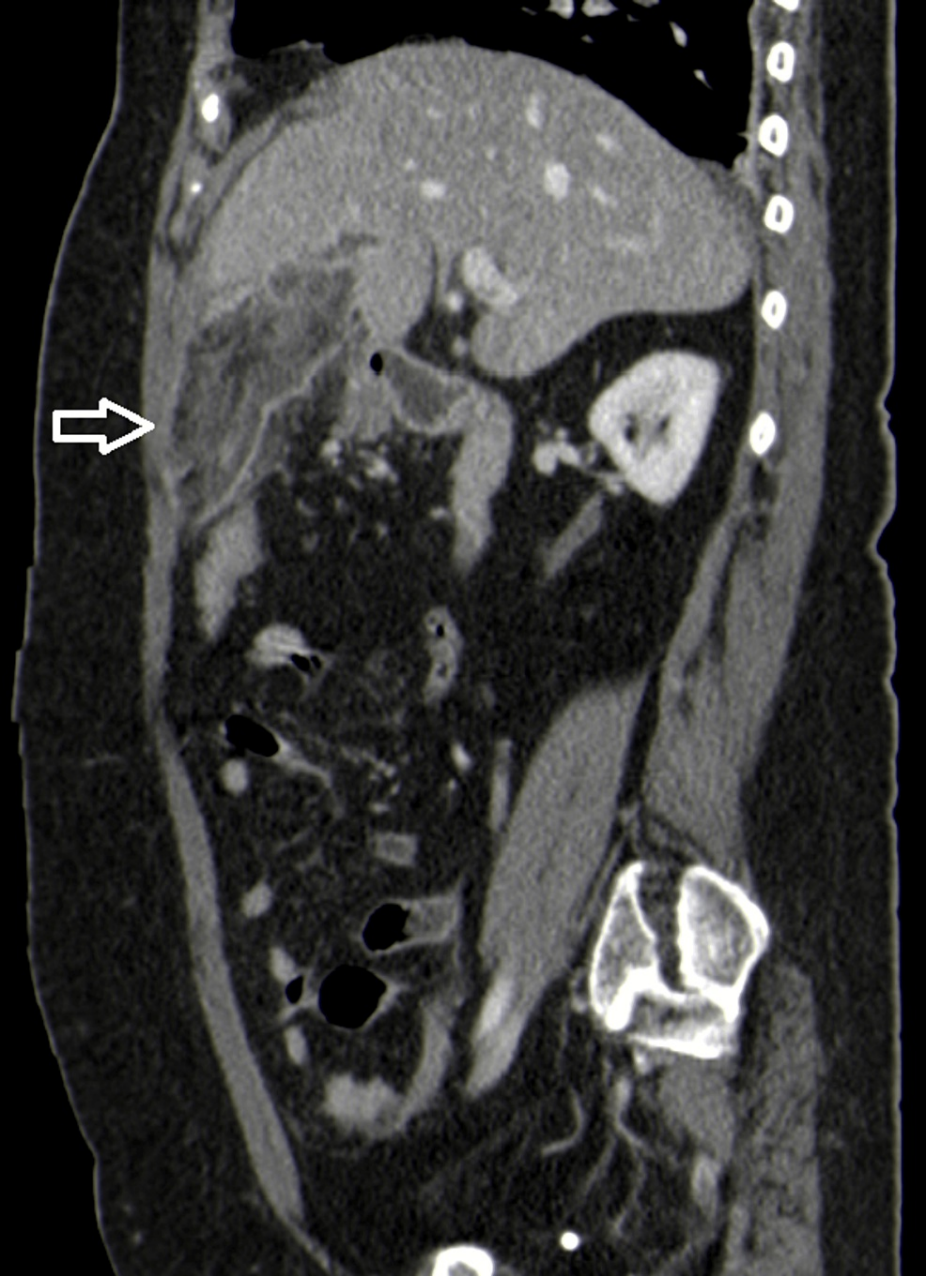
Sagittal computed tomography reconstruction showing the infarcted appendage (white arrow).
